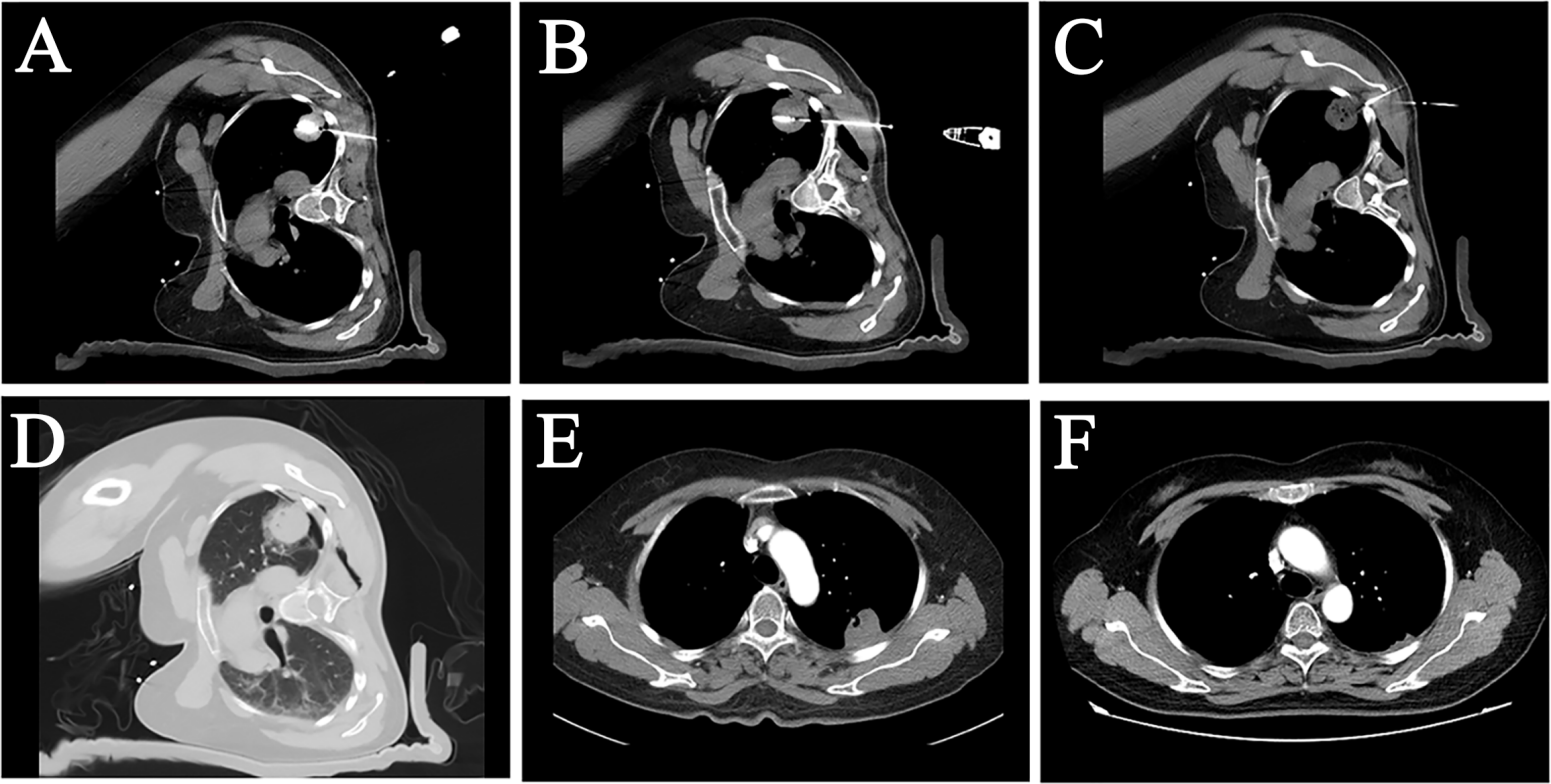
A female patient, 50 years old, with pulmonary metastasis from osteosarcoma, which was eradicated with an MWA procedure with LPA. (A, B) CT image showed a sizeable subpleural metastasis in the upper lobe of the patient’s left lung. Two MWA antennas were used on the patient at different layers to perform MWAof this metastatic tumor. (C) Local pleural anesthesia was used on the patient during the treatment. The tip of the anesthesia needle was placed in the subpleural area near the tumor. (D) An immediate postoperative CT scan showed a “halo sign” surrounded by a ground-glass density around the lesion. (E) A follow-up examination three months after surgery showed that the lesion was smaller than before, and no abnormal enhancement was seen inside the lesion. (F) Twelve months later, the tumor largely disappeared, suggesting that the pulmonary metastasis had achieved radical ablation.
